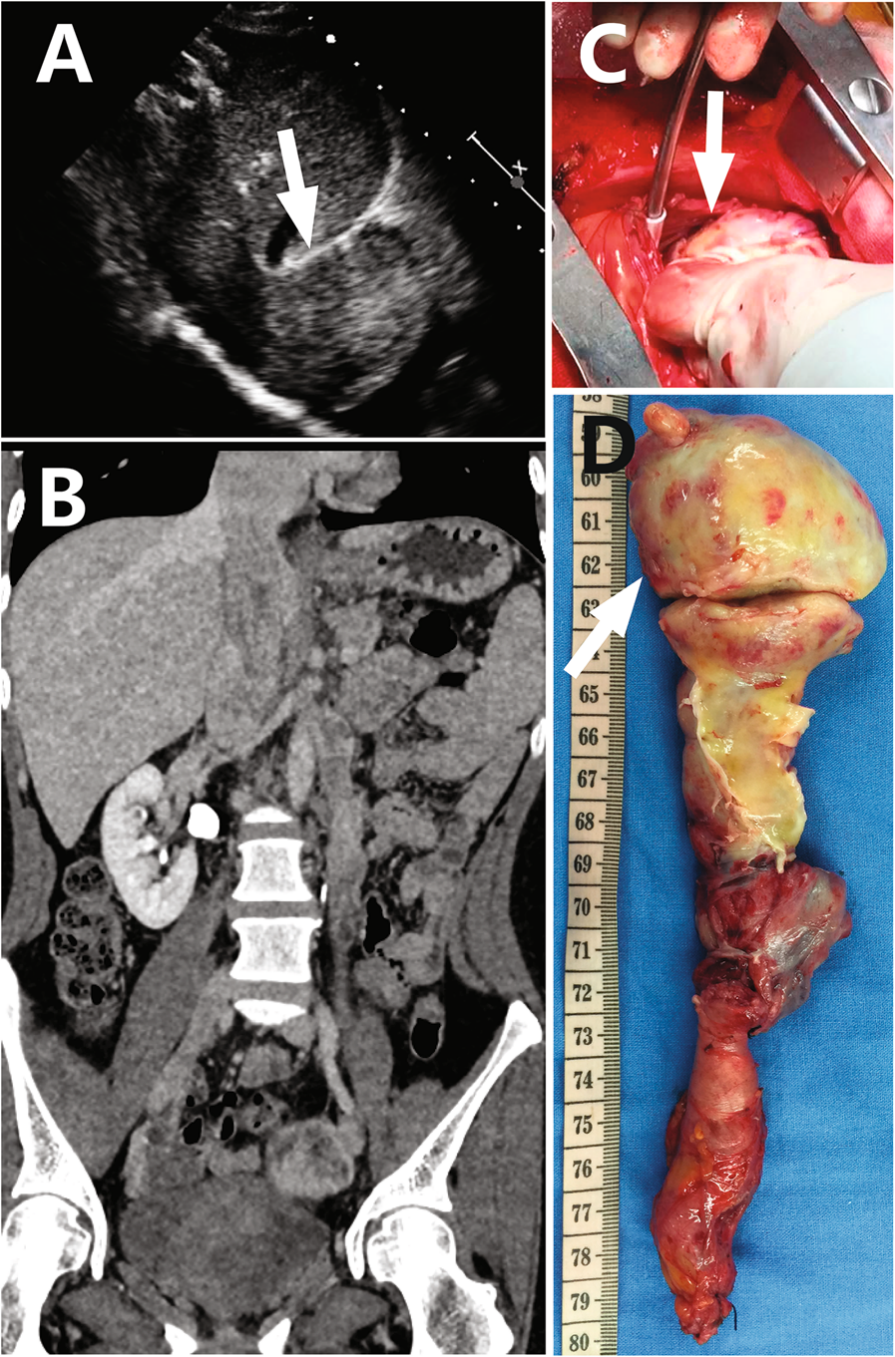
Echocardiogram (a) and venography (b) indicate that the over-dilated intracardiac head congests the chamber and is immobile. Tumor (c, d) massively adheres to the junction of the inferior vena cava with the right atrium and is resected through sterno-laparotomy. Arrow points to the site of tumor adherence
